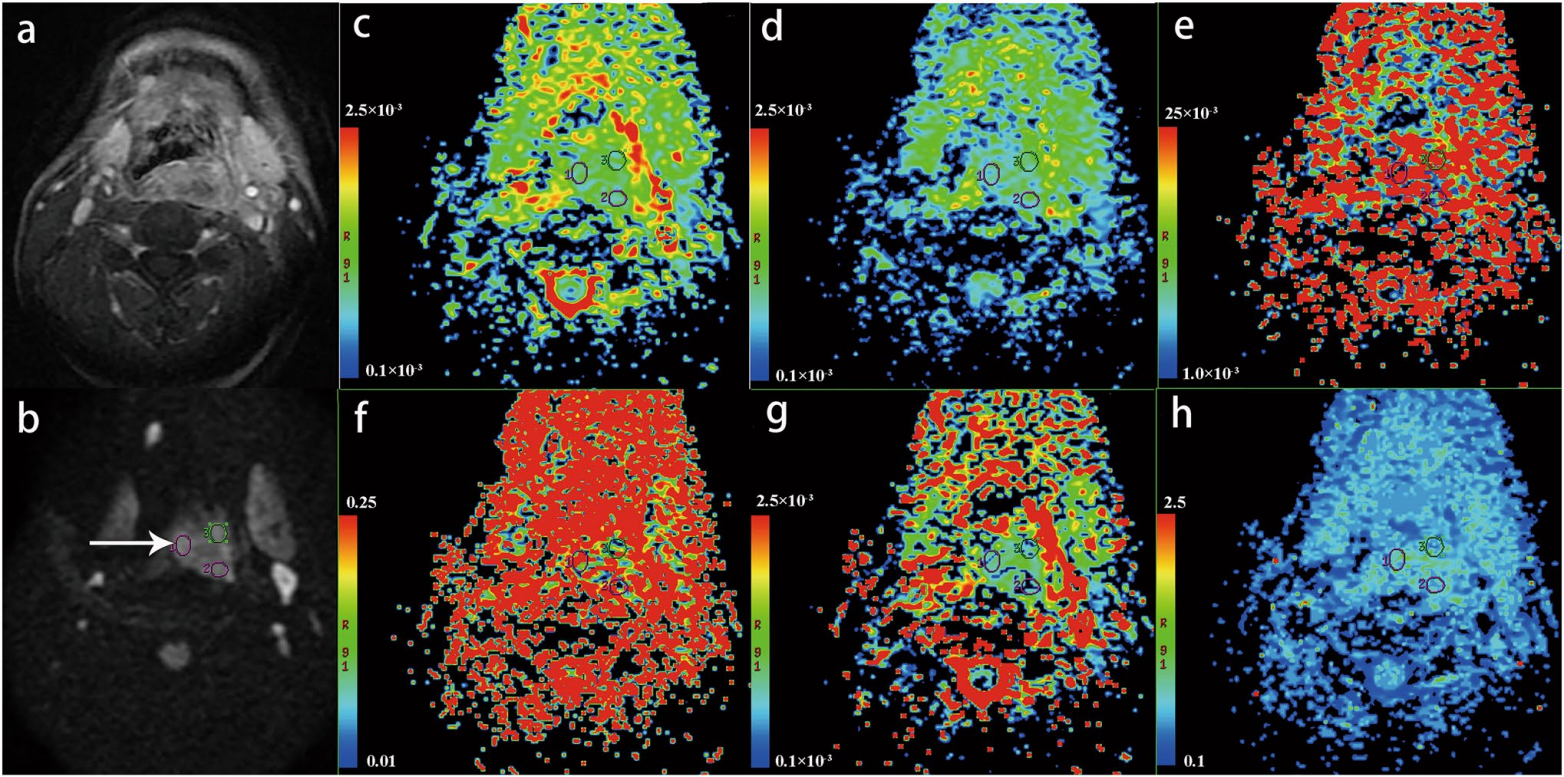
A 66-year-old man with hypopharyngeal squamous cell carcinoma is presented. Over 60 months of follow-up, no disease progression occurred. Axial contrast-enhanced T1-weighted image (a) shows a moderate enhanced primary tumor at posterior pharyngeal wall. IVIM image (b = 800 s/mm^2^ level) shows the mass has a slightly hyperintense signal (white arrow) (b). The three circles presented in the mass are the ROIs sketched artificially in the typical area of the largest level of the mass, and are applied to each functional map. ADC map (c), ADCmean = 0.936 × 10^−3^mm^2^/s, ADCmax = 0.1.41 × 10^−3^mm^2^/s, ADCmin = 0.695 × 10^−3^mm^2^/s, ADCrange = 0.845 × 10^−3^mm^2^/s; D = 1.01 × 10^−3^mm^2^/s (d), D* = 29.6 × 10^−3^mm^2^/s (e), *f* = 48.3% (f), DDC = 0.619 × 10^−3^mm^2^/s (g) and α = 0.751 (h) maps are shown.
